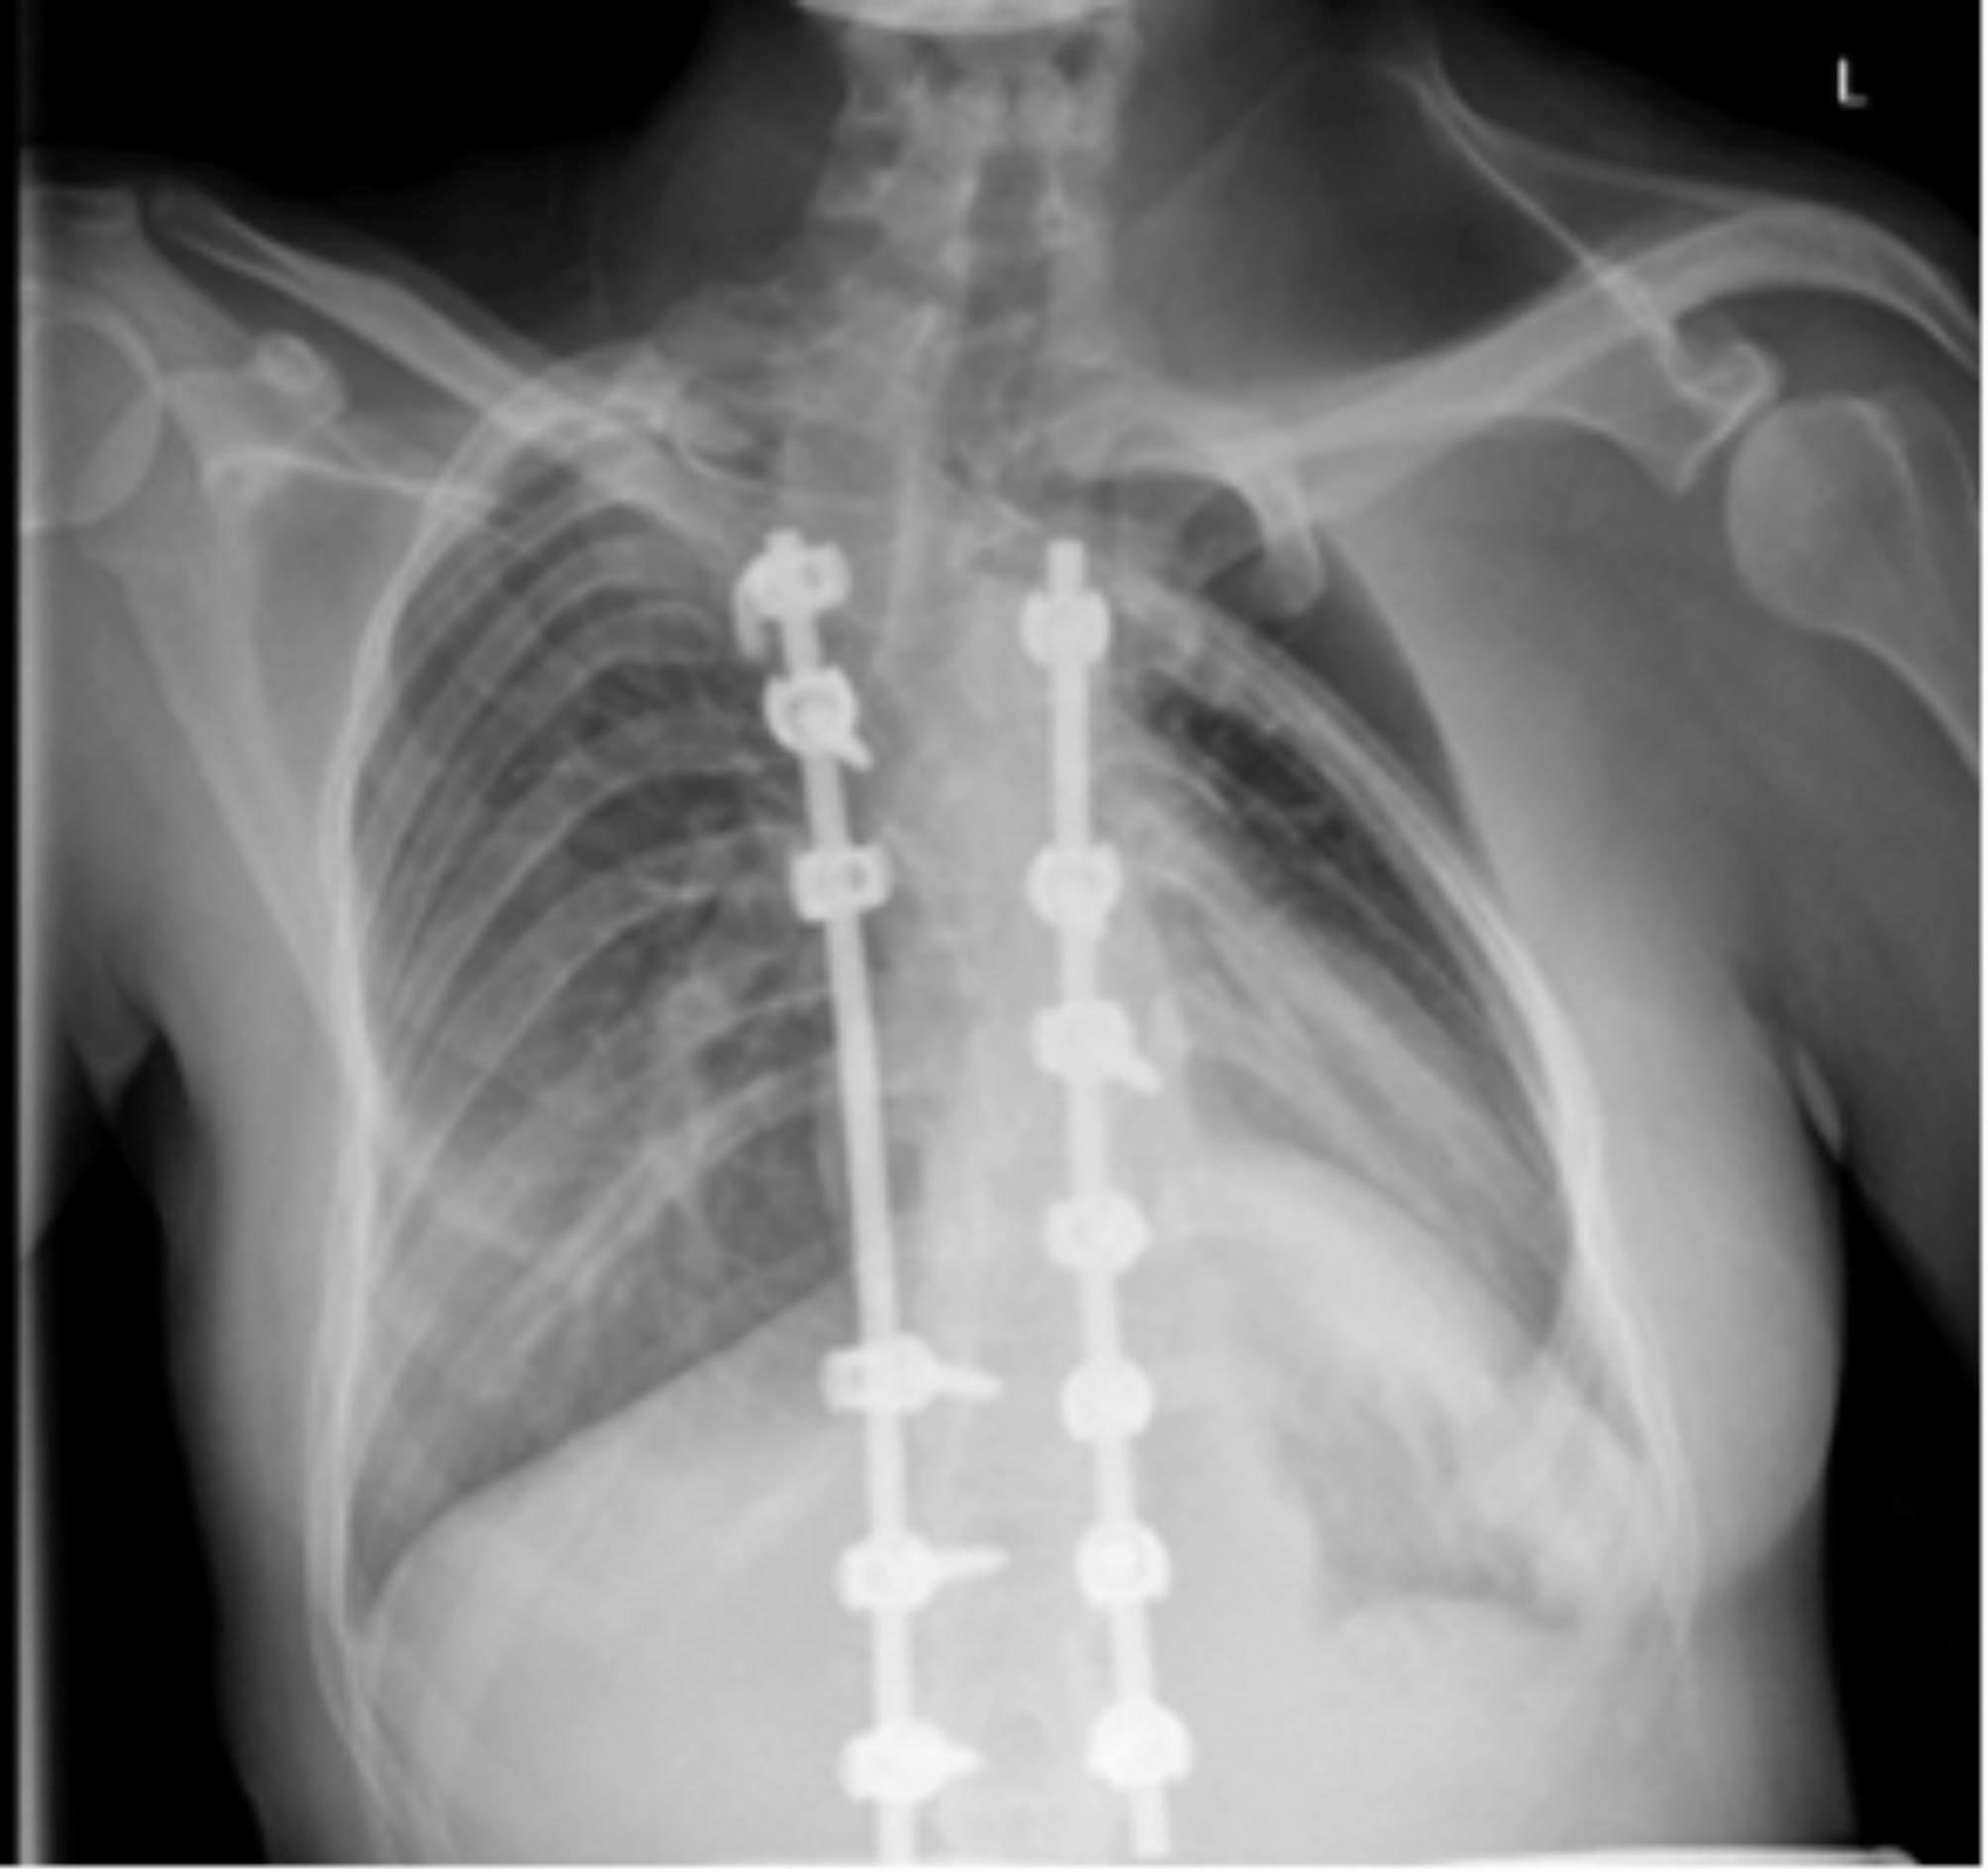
Chest x-ray showing displacement of the left scapula and diaphragm
